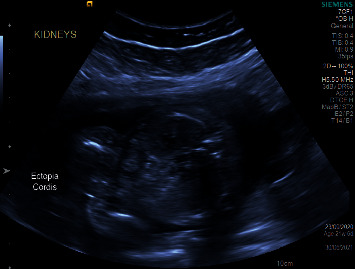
Ultrasound image showing both the kidneys and ectopia cordis.
